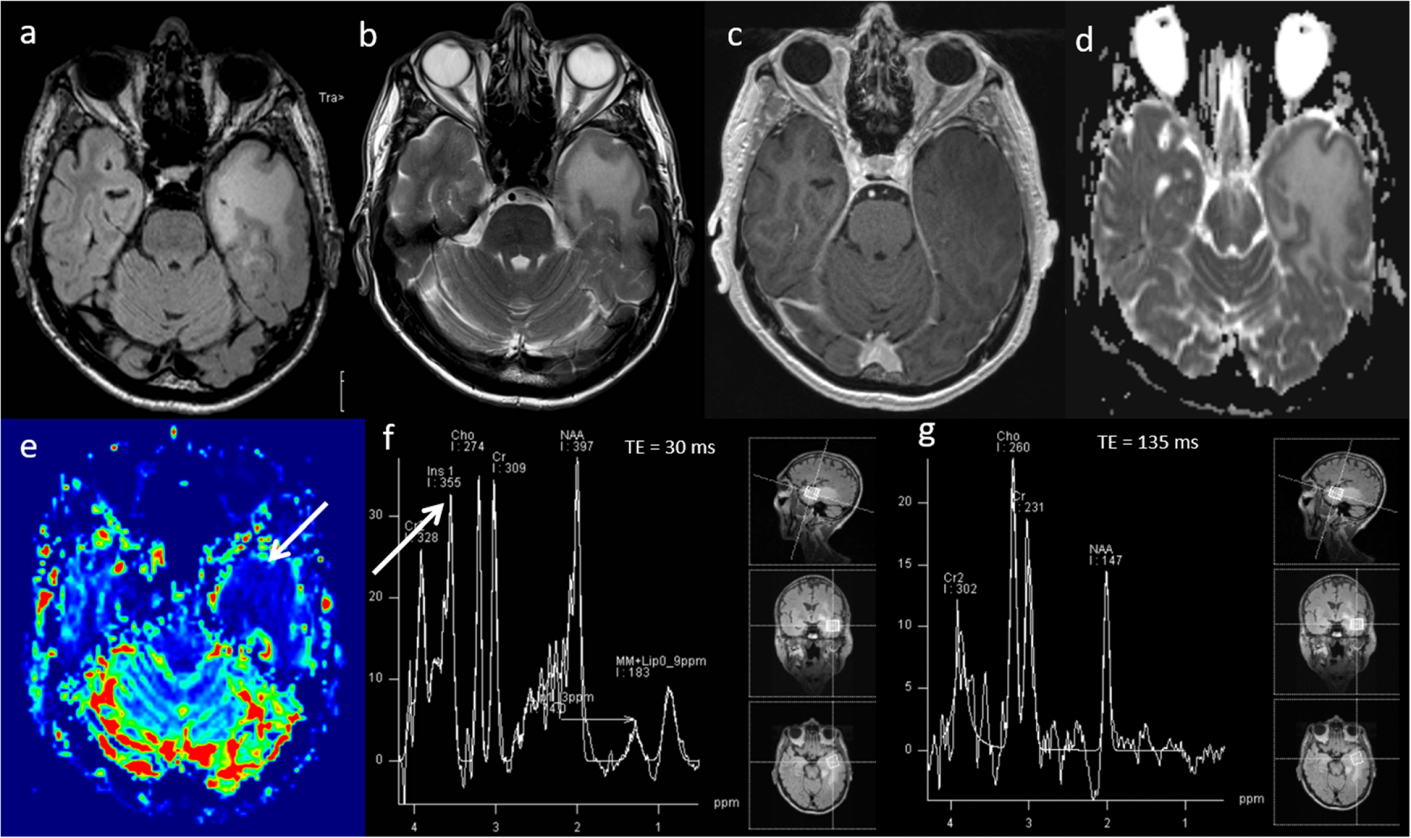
Low-grade glioma. Conventional MRI: (a) FLAIR, (b) T2W and (c) post-contrast T1W sequences show a diffuse abnormality in the left temporal lobe without contrast enhancement. d ADC map shows high ADC throughout the lesion (1300 × 10^−6^mm^2^s^−1^). e PWI shows low perfusion throughout the lesion (arrow) compared to normal-appearing white matter, and (f, g) MRS (TE 30 ms) shows slightly raised Cho/Cr ratio (1.0), slightly low NAA/Cr (1.1) and very high mI/Cr ratio (0.9, arrow). Lipid or lactate peaks are not significantly elevated. Multiparametric MRI appearances suggest no evidence of dedifferentiation. Stable appearances have been seen on follow-up imaging for over five years, confirming the lesion’s low-grade nature
